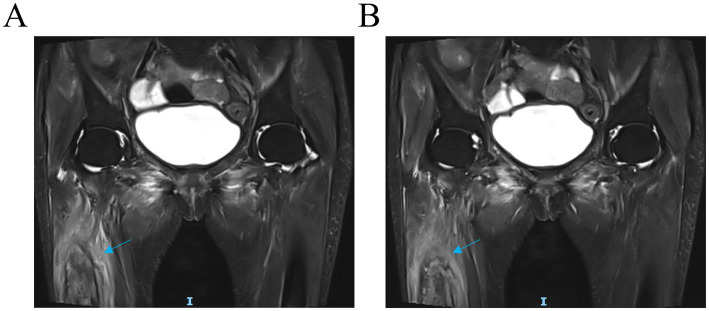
MRI of the patient’s knee and hip joints (A,B). The noncontrast MRI scan demonstrated a patchy area of high signal intensity on fat-suppressed sequences within the femoral shaft, accompanied with localized cortical bone destruction and a possible periosteal reaction (blue arrow).
